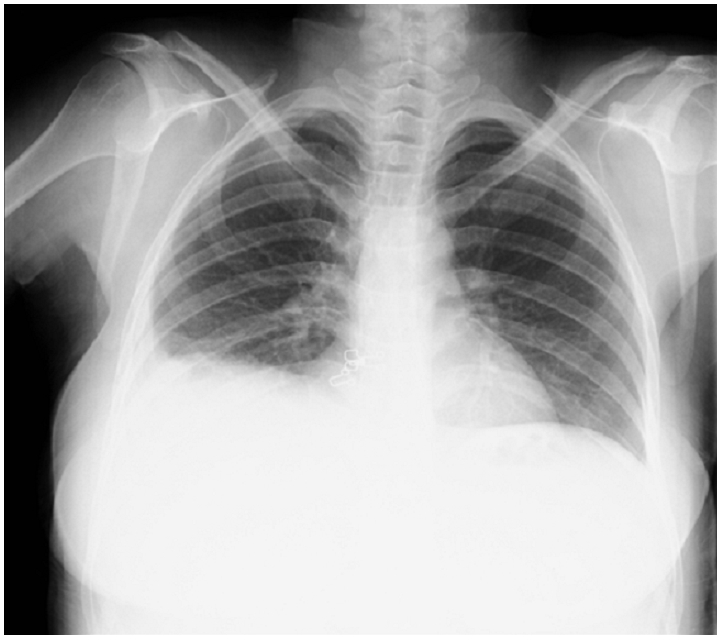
chest X-ray showing right pleural effusion in a patient with ovarian hyperstimulation syndrome in a spontaneous pregnancy with invasive mole
